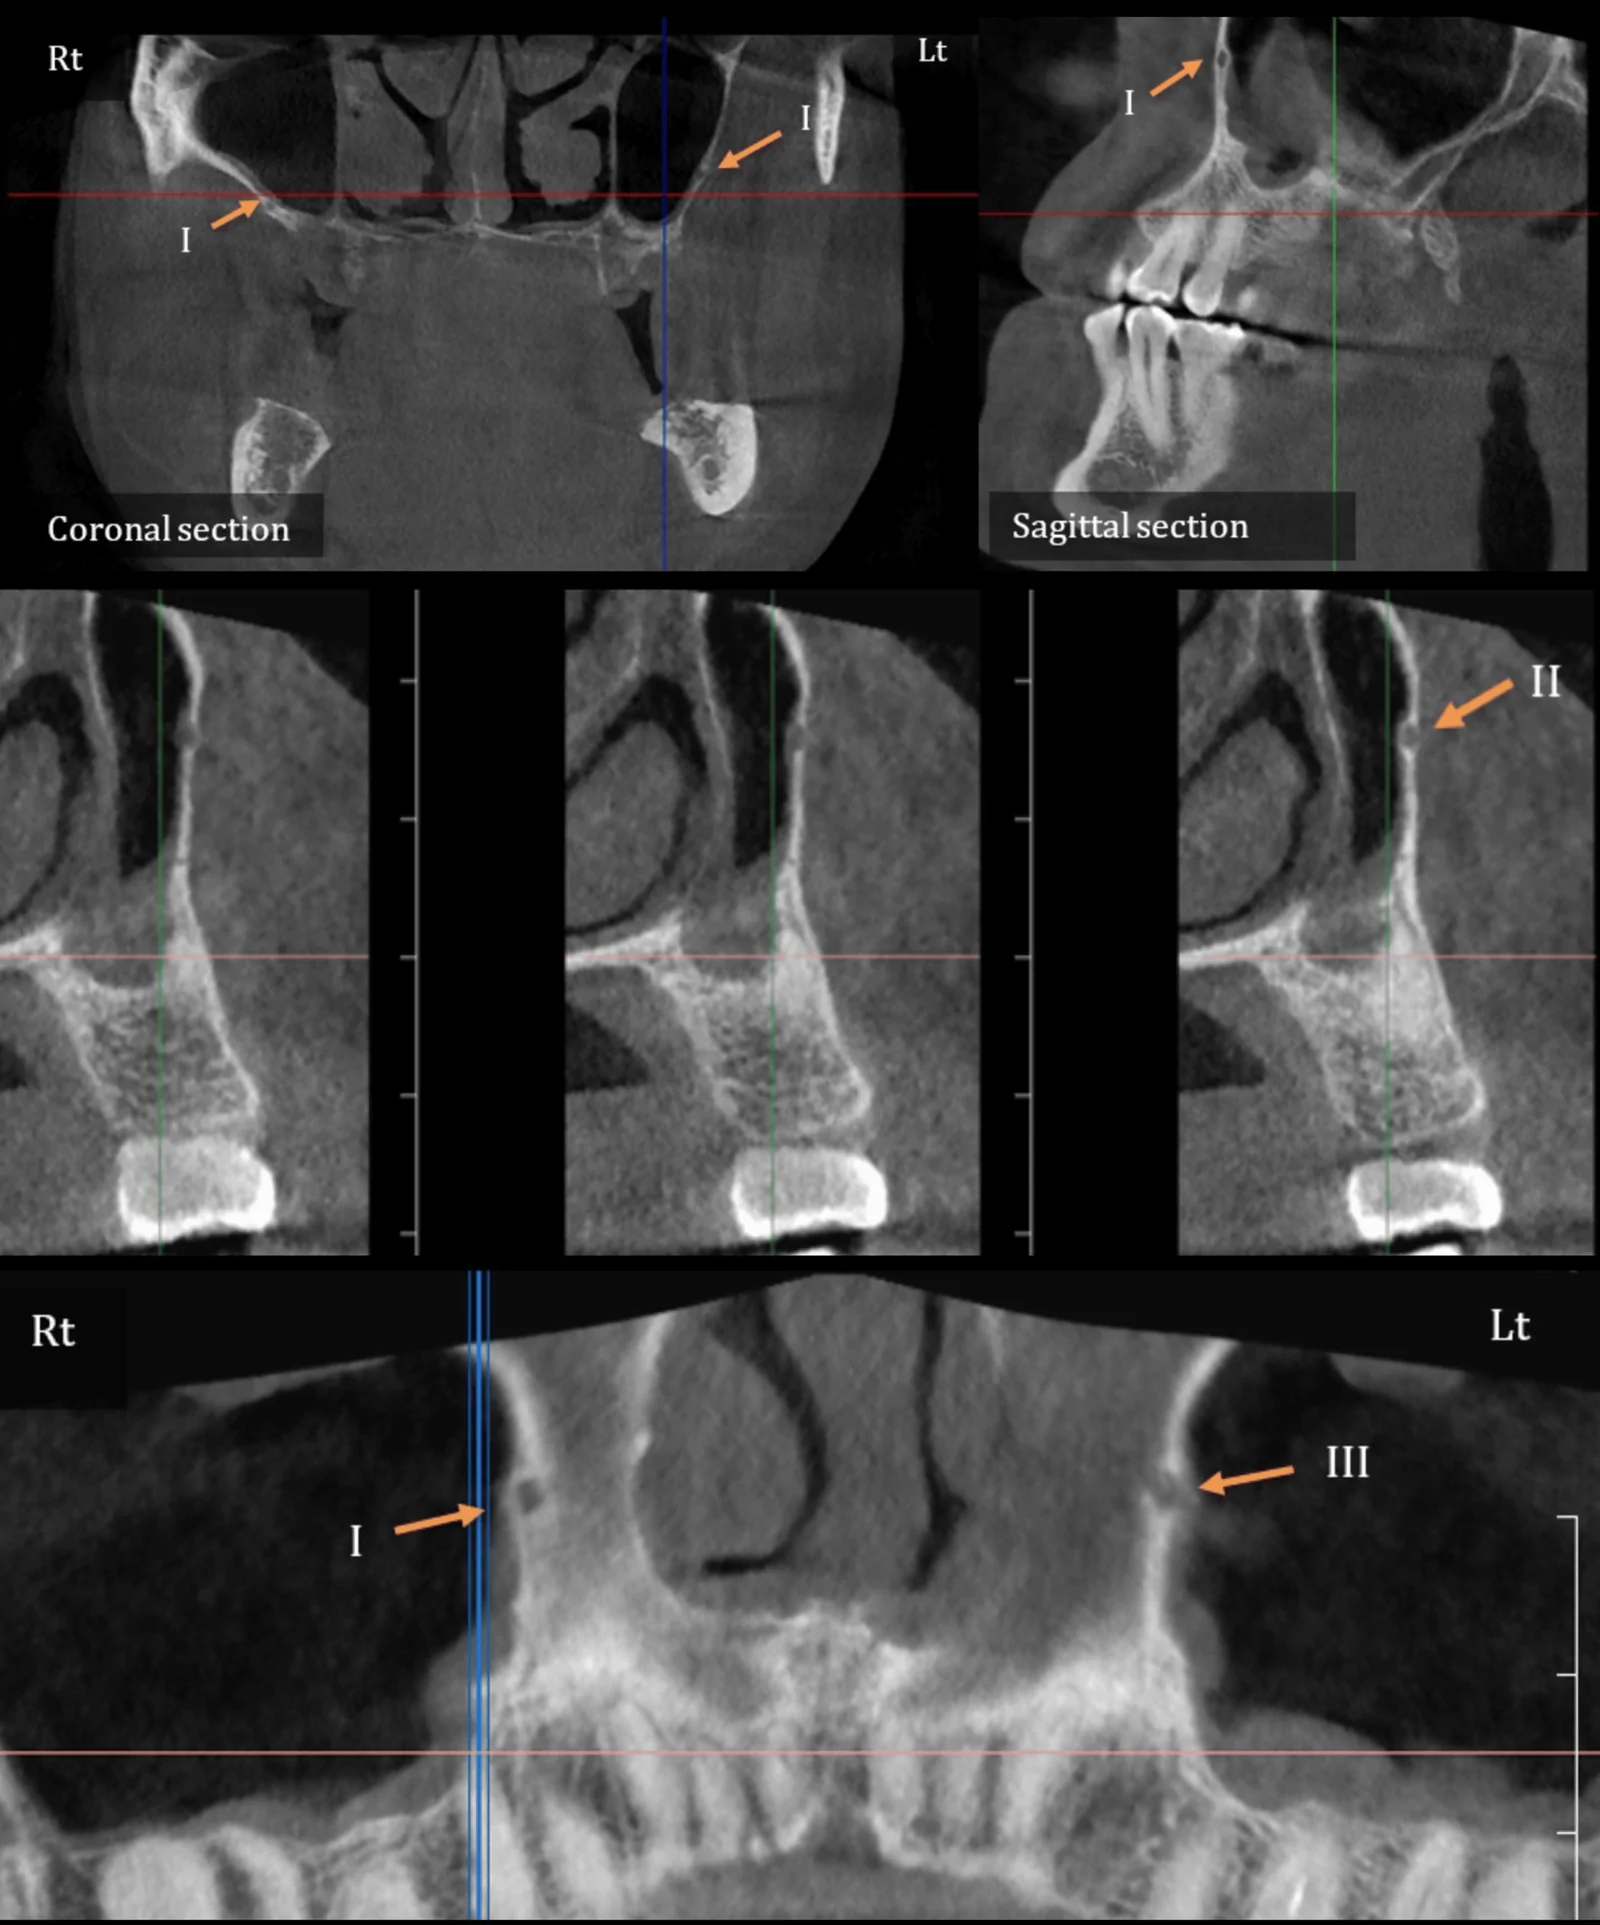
Different variations of the PSAA in coronal, sagittal, and curved sections (I, Intraosseous; II, Intrasinus; III, Superficial). CBCT images in coronal, sagittal, and curved sections demonstrating variations in the course of the posterior superior alveolar artery (PSAA). The PSAA is classified based on its relationship to the lateral wall of the maxillary sinus as (I) intraosseous, (II) intrasinus, and (III) superficial. Arrows indicate the location of the artery in each section.
